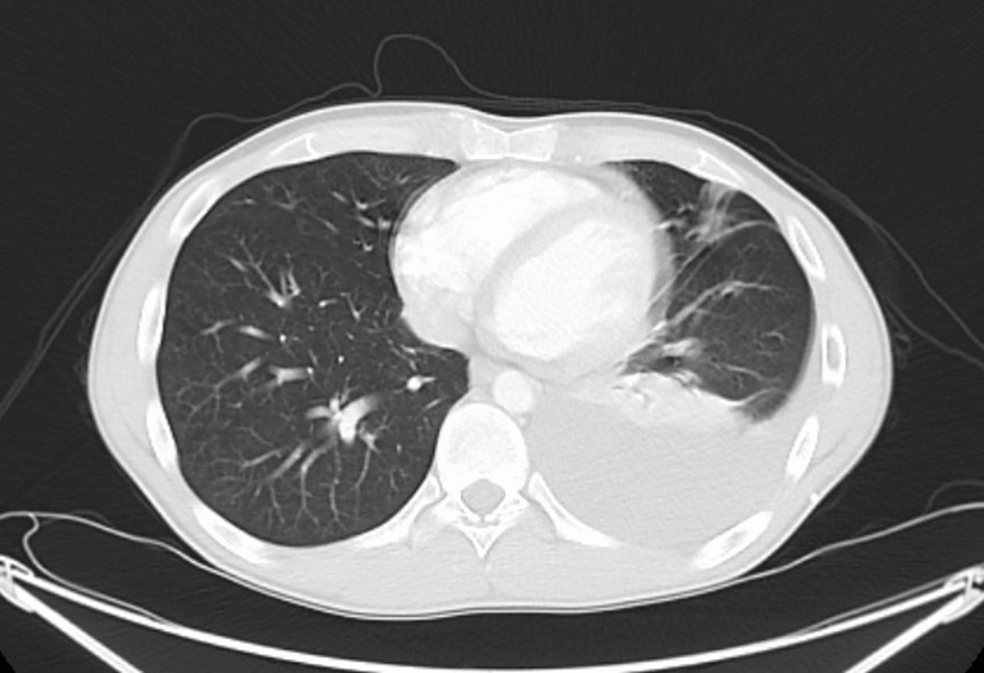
CT scan of the chest showing left-sided pleural effusion. CT, computed tomography
